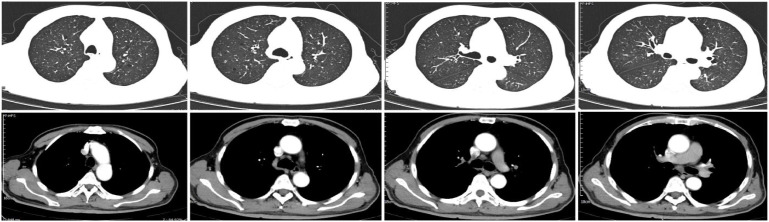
After 4 cycles of neoadjuvant therapy, follow-up contrast-enhanced chest CT showed that following neoadjuvant treatment for squamous cell carcinoma of the right upper lobe, the primary lesion was significantly reduced in size, and obstructive pneumonia was almost completely resolved.
